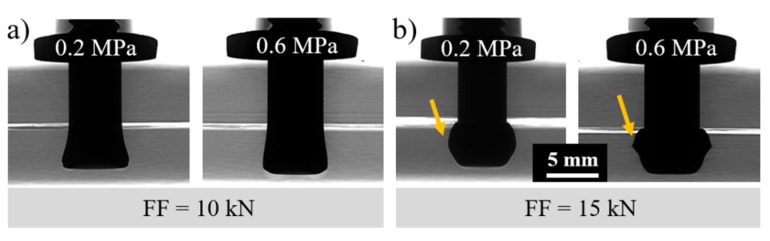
X-ray micro-computed tomography analysis of the joints produced with (a) low (10 kN) and (b) high (15 kN) friction forces, as well as the influence of clamping pressure on the rivet tip shape. (Joining condition—RS: 15,000 rpm, DF: 7.5 mm).
